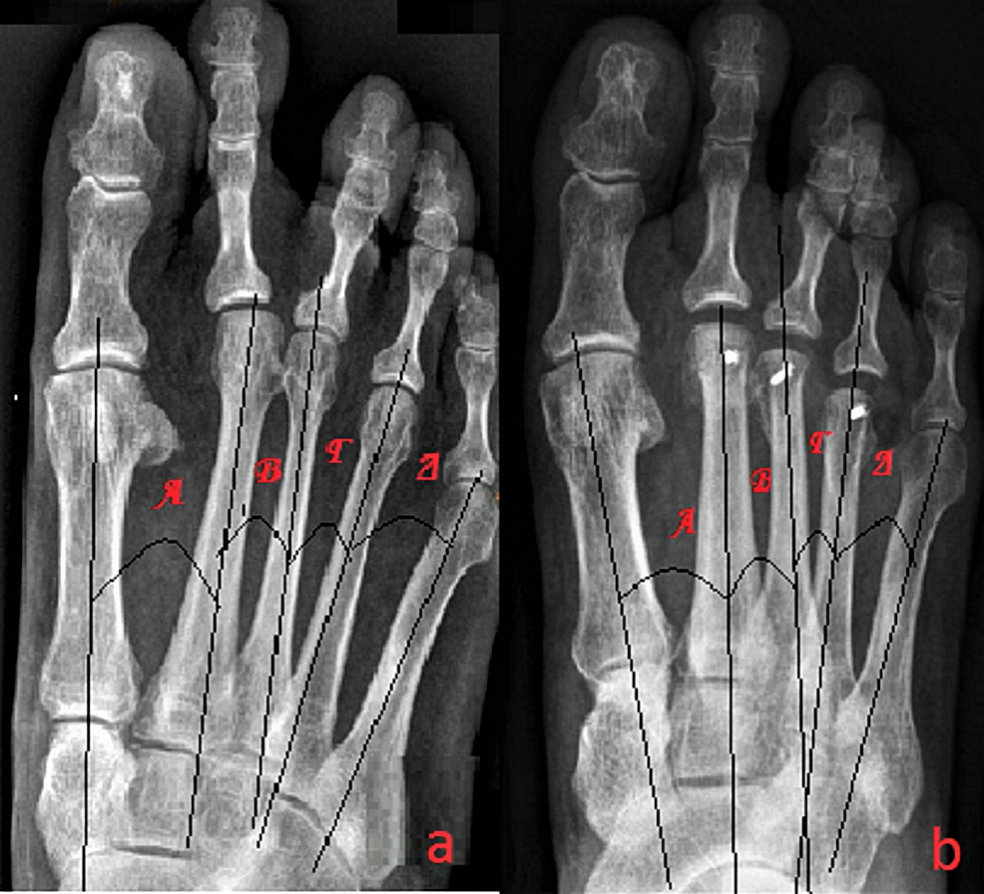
A 47-year-old patient with biomechanical metatarsalgia due to first ray insufficiency and concomitant second ray syndrome. In order to avoid possible deviation due to the loss of absolute stability at the frontal level, measurements of the angular relationship between the longitudinal axes of the metatarsals were determined. (a) Preoperative anteroposterior weight-bearing X-ray and (b) postoperative preoperative anteroposterior weight-bearing X-ray.
